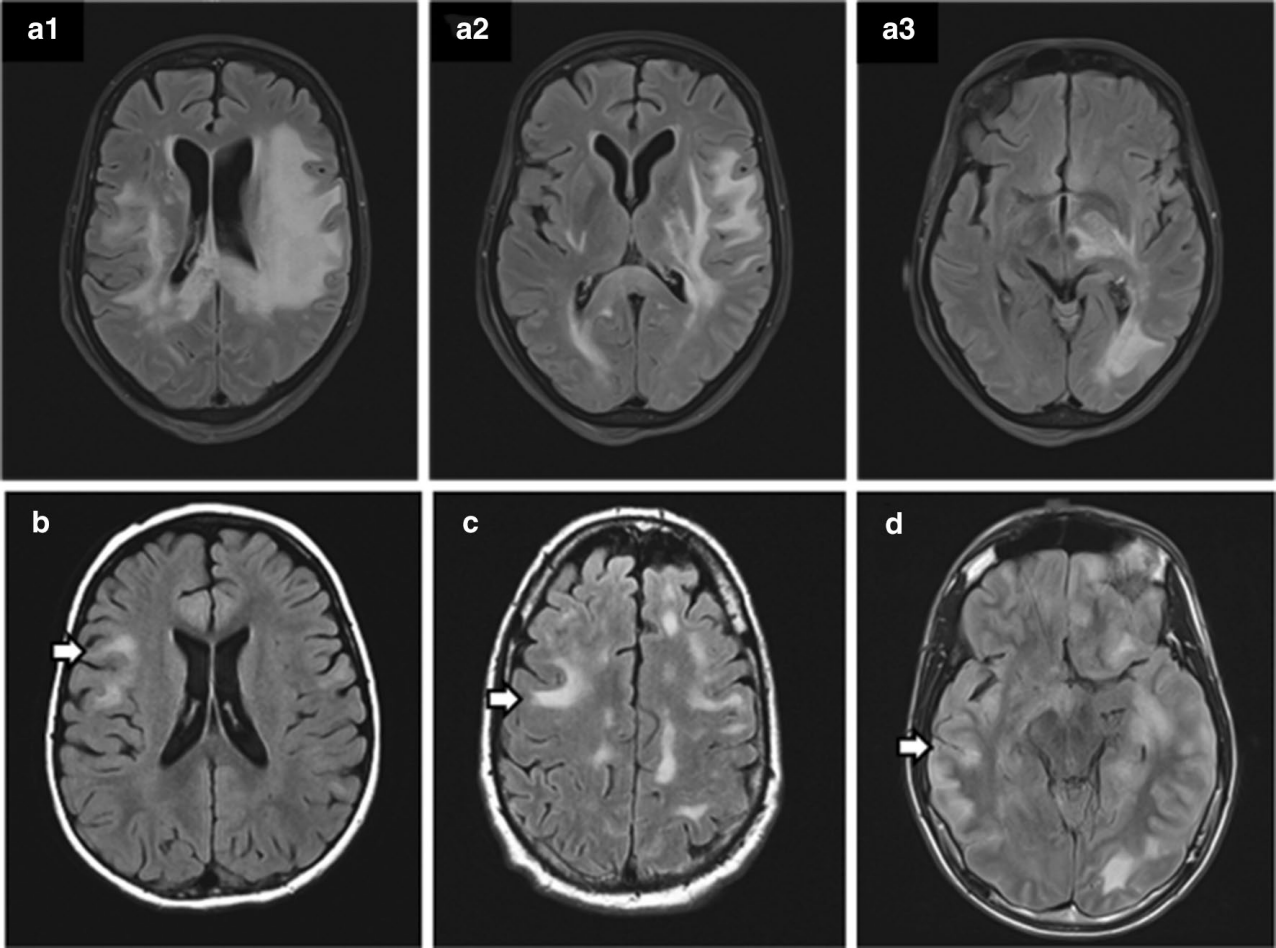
MRI findings in patients with MOG-associated encephalomyelitis. a1–a3 Brain MRI from autopsy patient 1, obtained on day 40 after symptom onset, shows extensive, confluent T2 abnormalities involving the subcortical white matter of the frontal, temporal, and occipital lobe, bilaterally, with contrast enhancement in one fronto-parietal lesion (not shown) and extension into deep grey matter. b–d MRI of 3 MOGAD biopsied cases just prior to surgery with the arrow reflecting the site of biopsy
